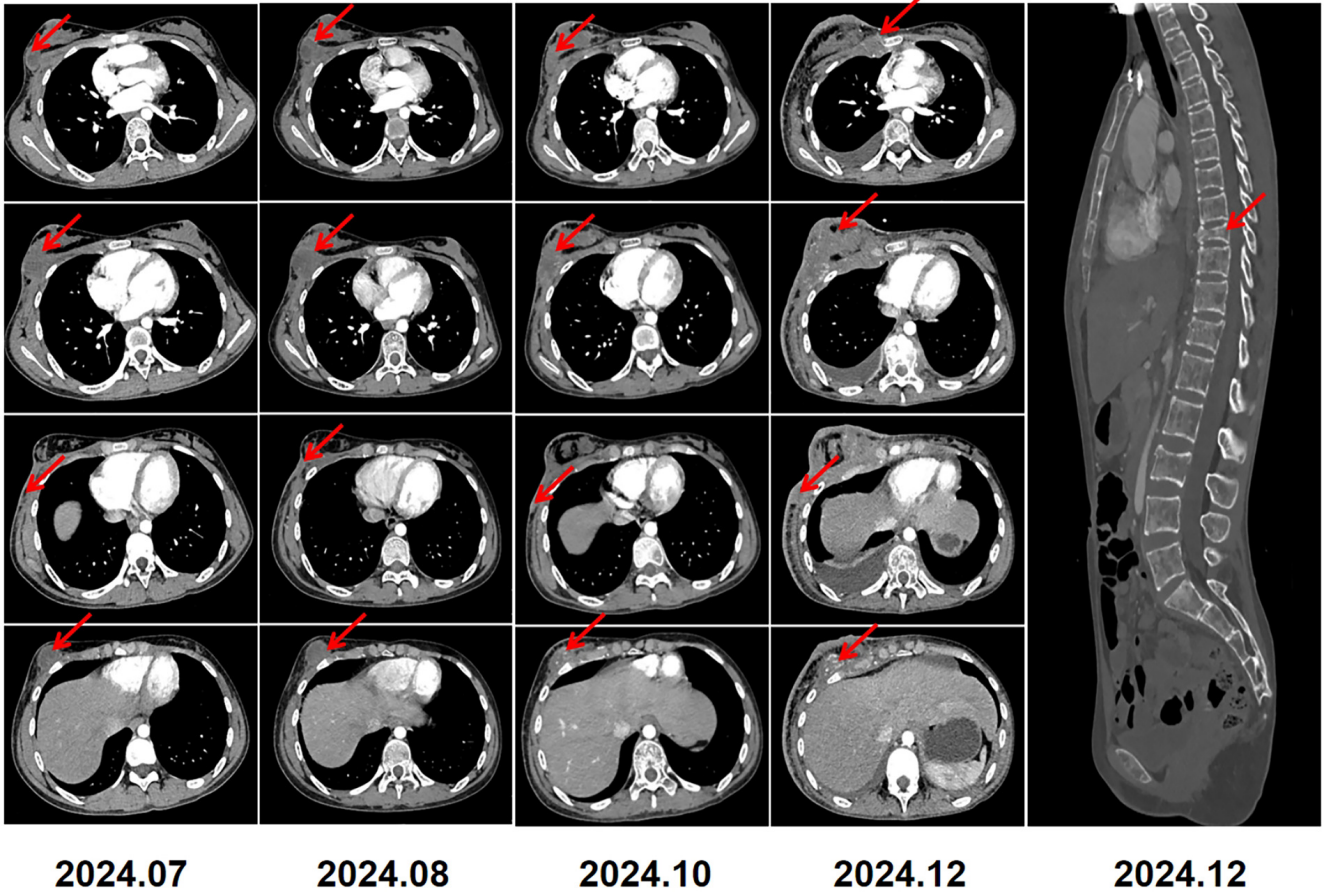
2024.7–2024.12: serial CT imaging demonstrated tumor recurrence involving the right breast, chest wall, and abdominal wall. Diffuse thickening of the overlying right breast skin was noted. Bilateral pleural effusions, small in amount, were present. Imaging also revealed diffuse lytic bone destruction with a pathological compression fracture of the T9 vertebra.
